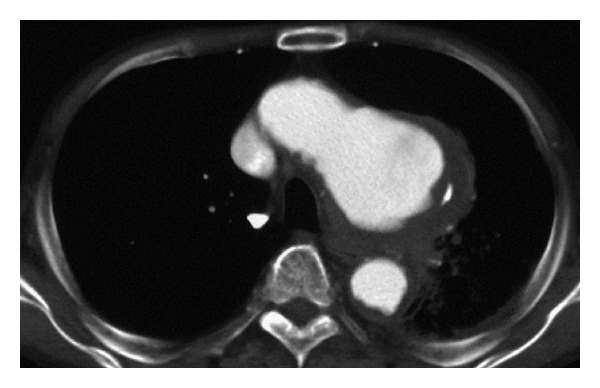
Transverse contrast-enhanced CT of the chest showing an enlarged aortic saccular aneurysm, protruding downward, surrounded by a hematoma in the aortopulmonary window.
